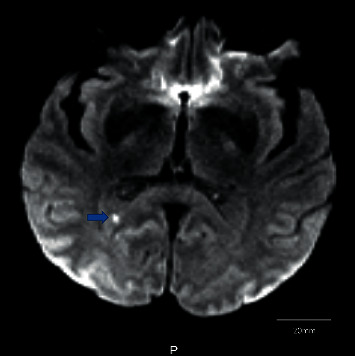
MRI of brain with contrast showing acute-to-subacute 5.3 mm embolic brain infarct (blue arrow).
